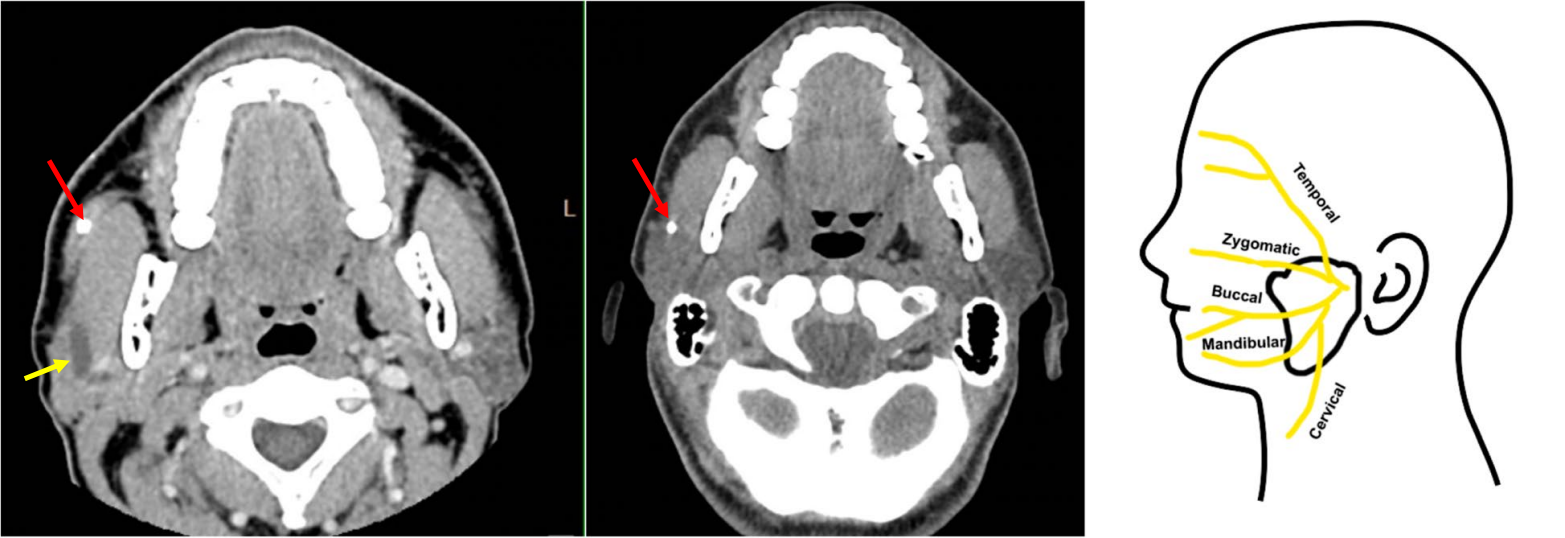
First two panels: CT Head, third panel: anatomical representation of branches. On the left showing CT neck from current admission and in the middle showing CT neck from 6 months ago. Red arrows point to stone location. Yellow arrow points to dilated Stensen’s duct
